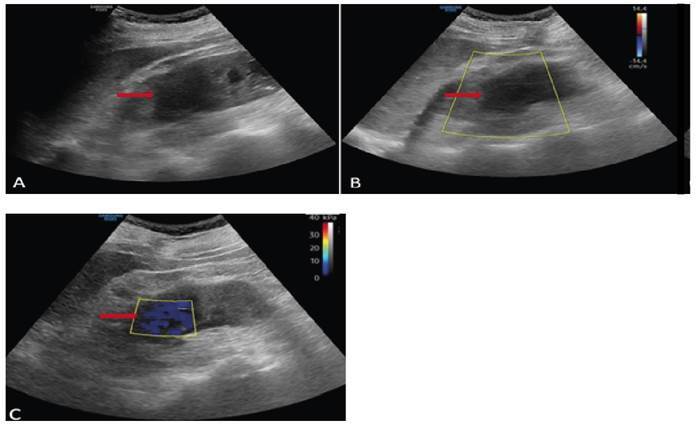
Longitudinal gray scale (A), color Doppler (B) ultrasound, and shear-wave elastography (C) images of the lesion in the right kidney. Doppler ultrasonography shows a hypoperfused lesion in the renal parenchyma of the right kidney. The sonoelastography images showed the soft stromal structures (red arrows).
